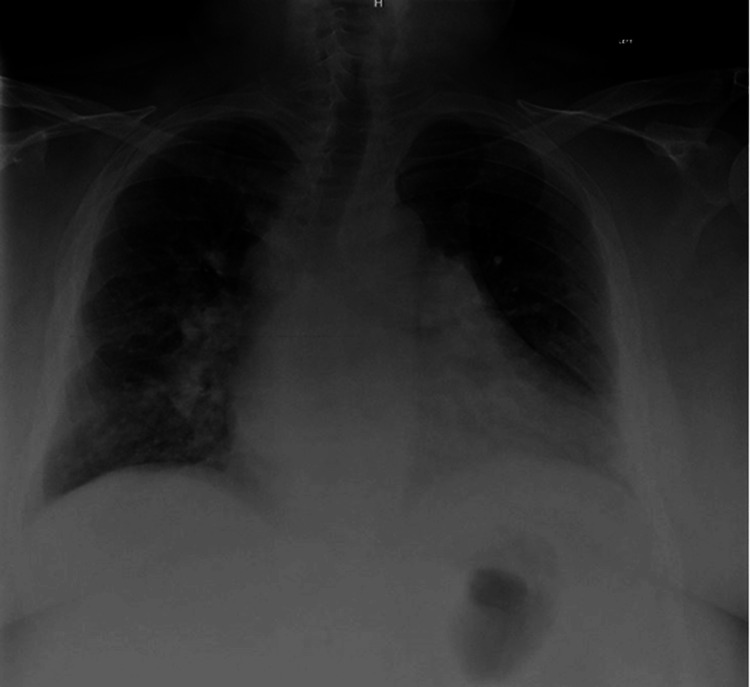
Chest X-ray Chest X-ray demonstrated stable cardiomegaly with no acute process.
